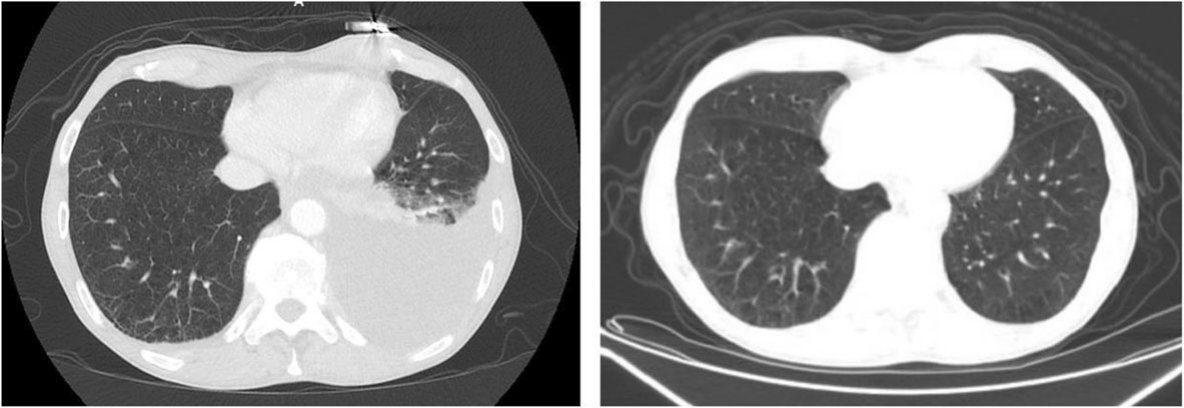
Chest CT demonstrated left-sided pleural effusion on initial presentation and complete resolution status post ibrutinib treatment on follow-up chest CT imaging. *CT* computed tomography, *PF* pleural fluid
